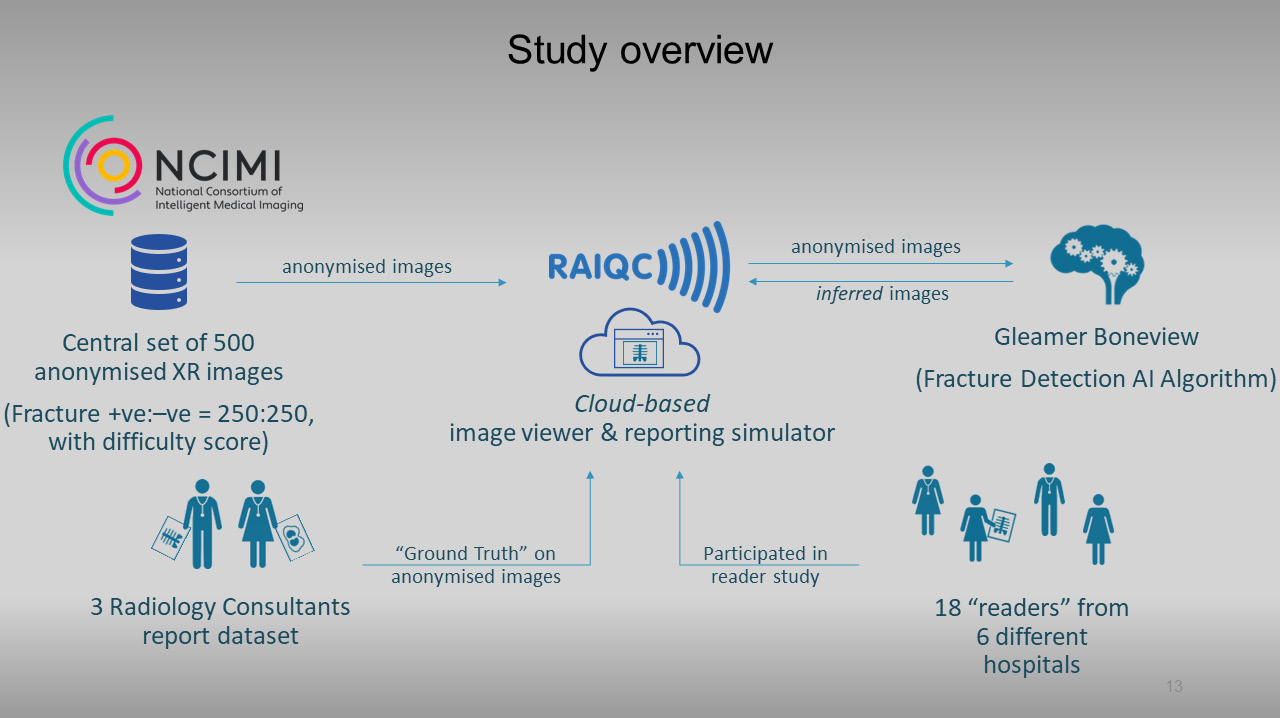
Artificial intelligence-assisted image analysis on the diagnostic accuracy of front-line clinicians in detecting fractures on plain X-rays study dataflows. AI, artificial intelligence; RAIQC, Report and Image Quality Control; XR, X-rays.
